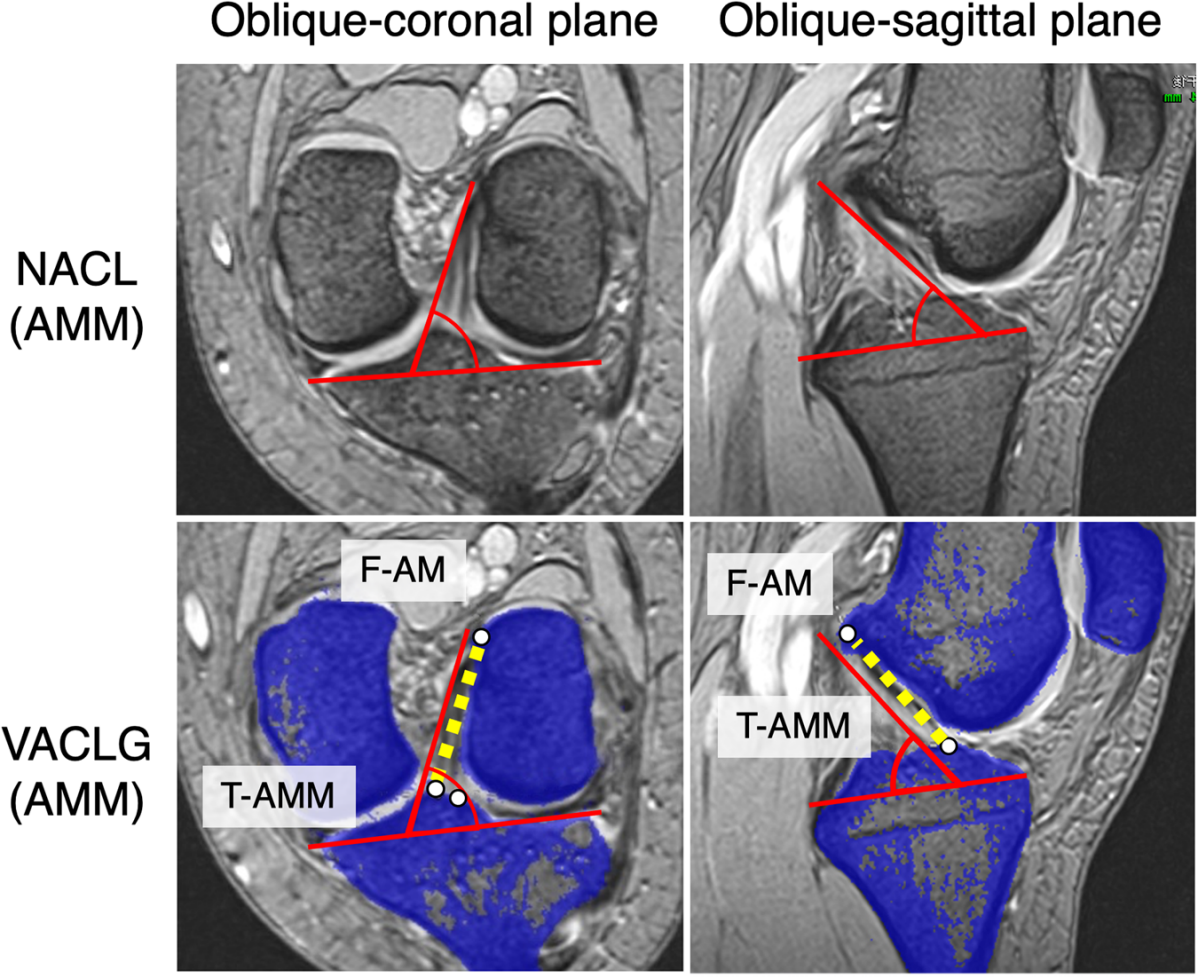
ACL‐tibial plateau angle (ACL‐TP angle) of the anteromedial bundle medial part (AMM) of the normal ACL (N‐ACL) and the virtual triple‐bundle ACL graft (VACLG) in MRI oblique‐coronal and sagittal planes. The ACL‐TP angle on the sagittal plane is the angle between the centre of the ACL (dashed line) and the approximating tibial plateau. The ACL‐TP angle in the oblique‐coronal is defined as the angle between the dashed line of the ACL and the midline of the lateral and medial tibiofemoral joint compartments. ACL, anterior cruciate ligament; MRI, magnetic resonance imaging.
